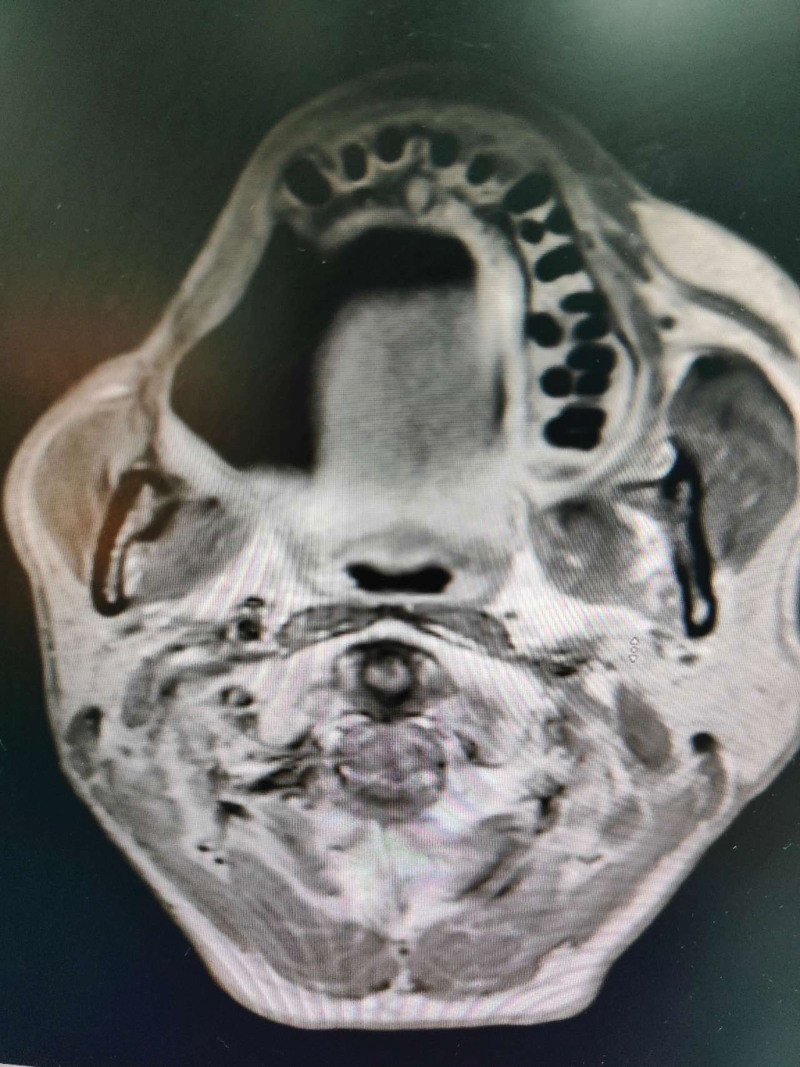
CT scan revealed a partial absence of the right mandible.
